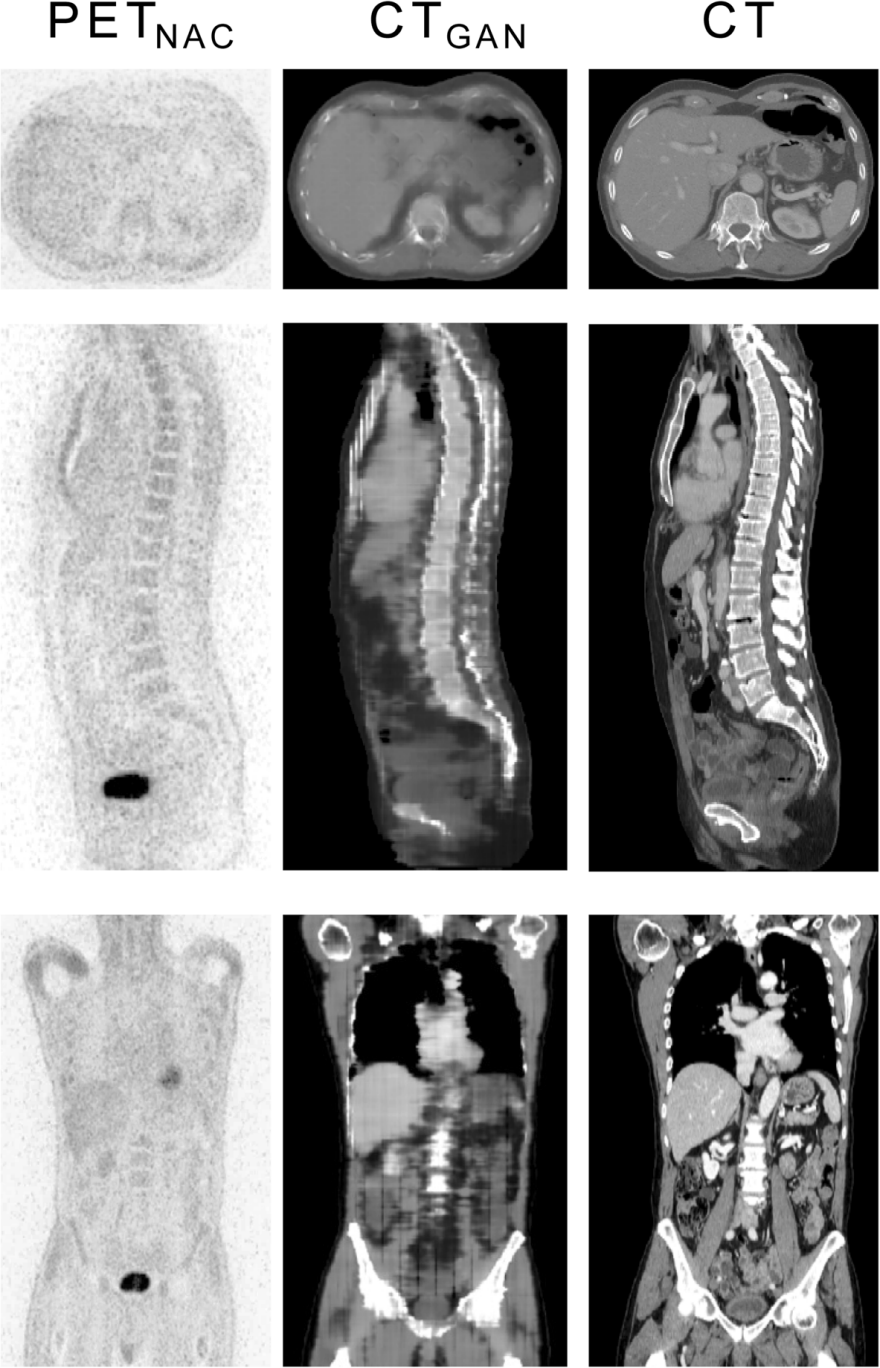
Representative data set showing non-attenuation-corrected [^18^F]FDG-PET (left), generated pseudo CT (middle), and acquired CT (right) in axial (top), sagittal (middle), and coronal (bottom) orientation. CT_GAN_ data—while capturing the coarse distribution of CT anatomy—showed blurring and step formation in *z*-direction on coronal and sagittal reconstruction as well as irregular depiction of anatomical details
